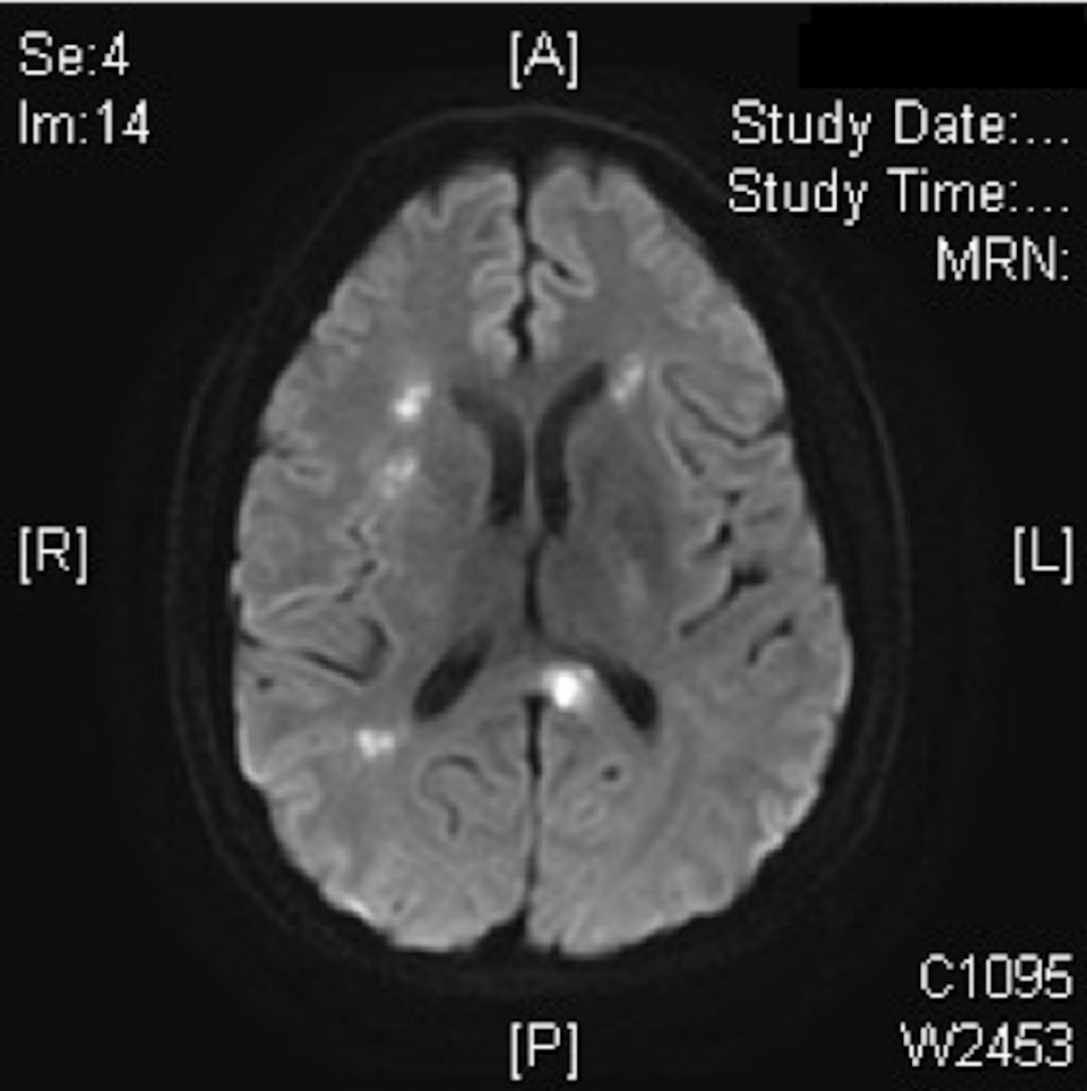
Hyperintensities on T2-weighted imaging located in the white matter, more frequent in the periventricular area
